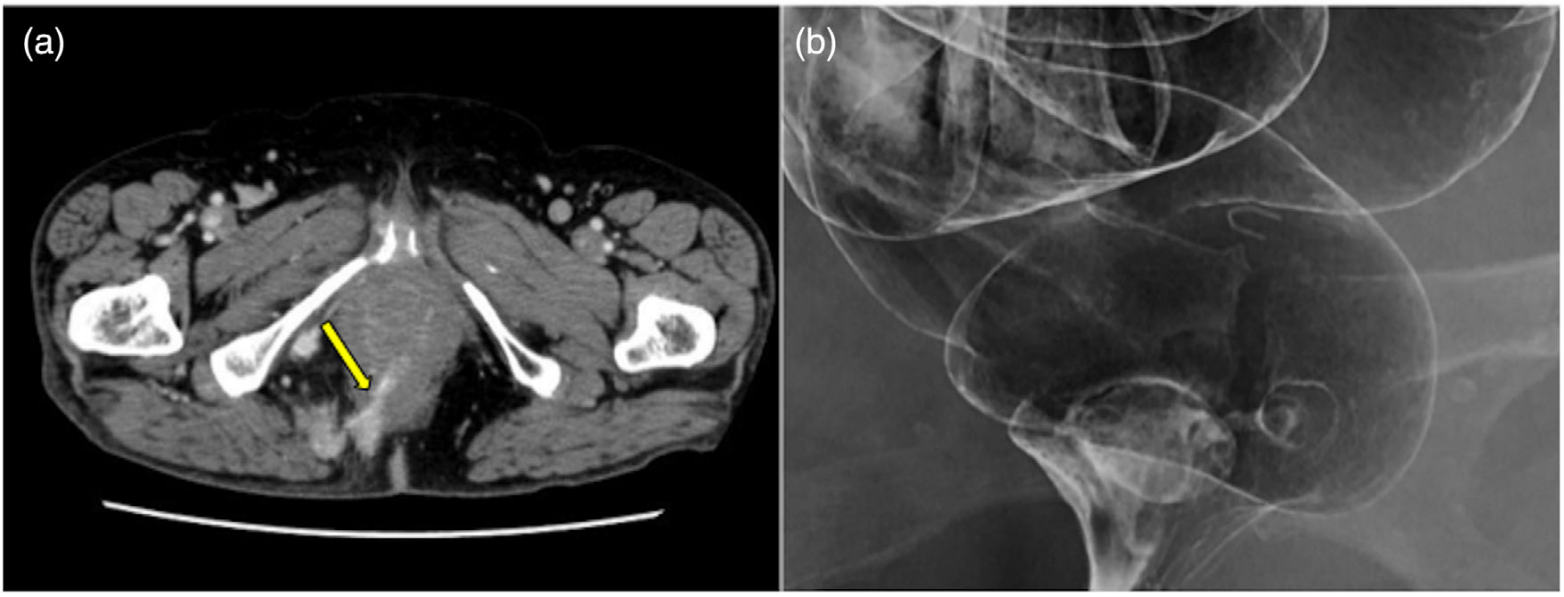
(a) Computed tomography showed a linear, high‐density area puncturing the vulva and rectum (yellow arrow). (b) Under barium enema examination to check for intestinal leakage or a fistula, there was not any leakage of contrast material through the lesion.
